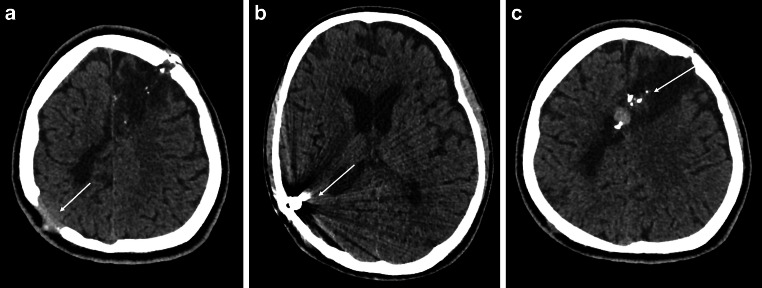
Non-enhanced cranial CT image in axial section of a diametrical shrapnel injury. a The entrance in the left frontal area, exit (*arrow*) in the right parietooccipital area with postcontusional parenchymal lesions. b Shrapnel near bone margins inside the brain parenchyma (*arrow*). c Bone fragments, that caused the injury of both ACA in the midline (*arrow*)
